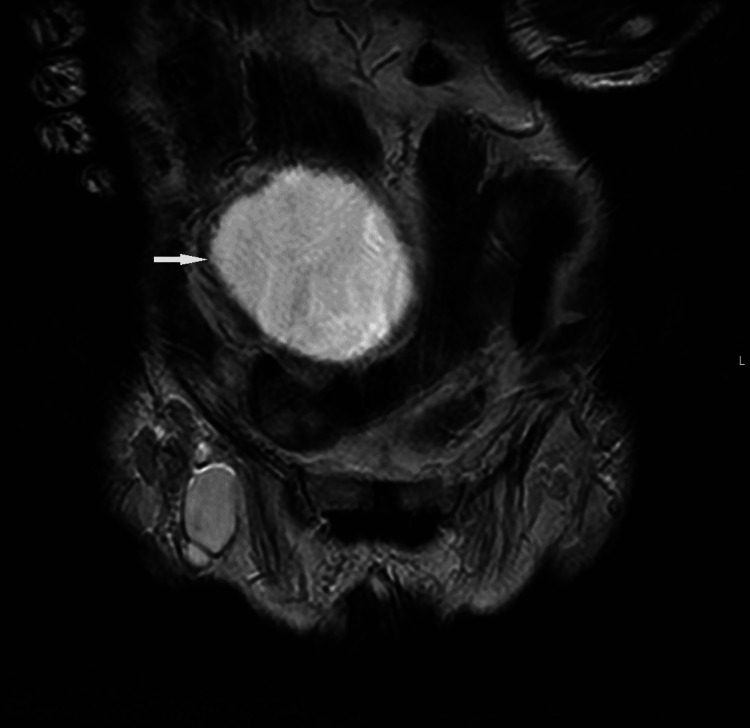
MRI Abdomen and Pelvis (Coronal View): Coronal view reveals a large simple seminal vesicle cyst with complex features
